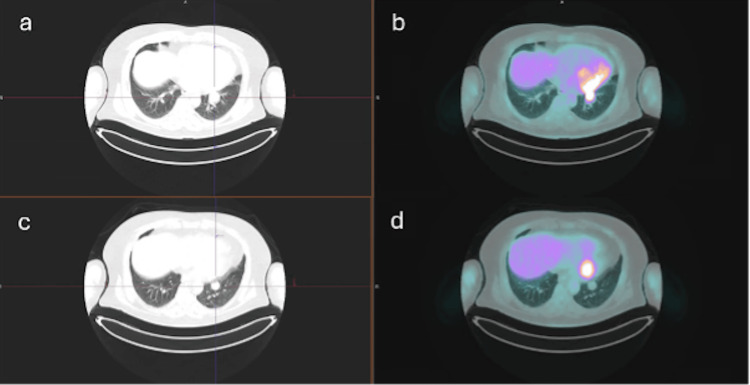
Axial CT and axial FDG-PET/CT images (a, b) demonstrating a hypermetabolic left basal pulmonary nodule, consistent with metastatic disease. Follow-up axial CT and axial FDG-PET/CT images obtained 3 months after the initial FDG-PET/CT (c, d) show a marked reduction in FDG uptake, with a near-complete metabolic response comparable to background activity.
